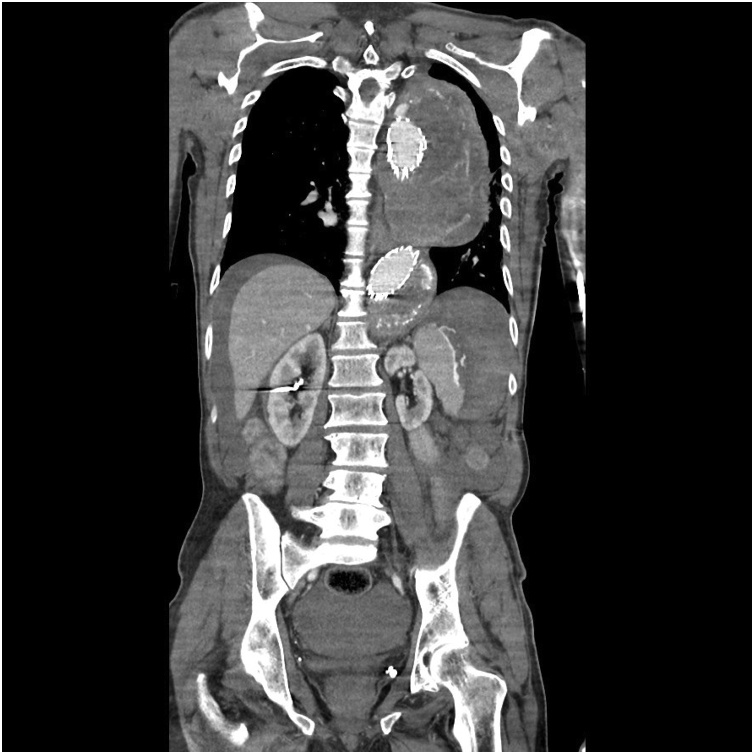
Sagittal image of computed tomography angiogram demonstrating splenic rupture, haemoperitoneum and remnant arterial aneurysm sac.
